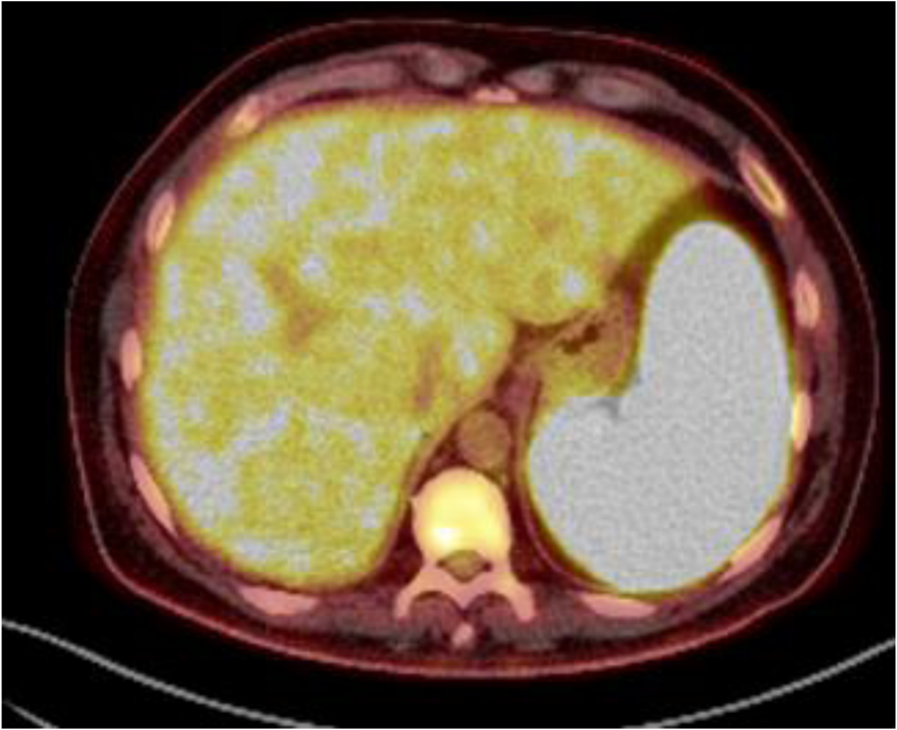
FDG-PET/TC. FDG-PET scans shows diffuse high uptake in the spleen (SUV máx: 13.26), liver, and bone marrow
